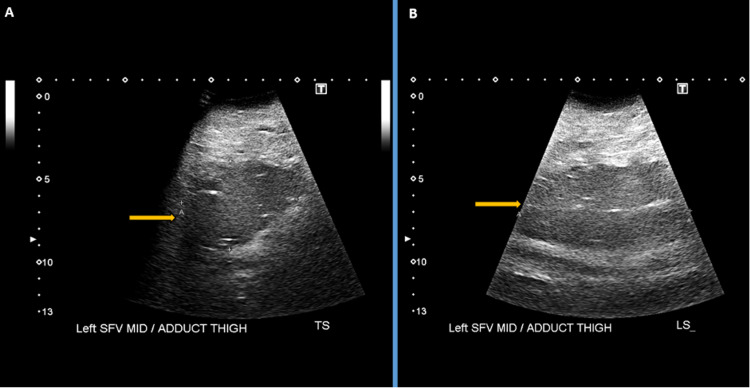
Pre-operative greyscale ultrasound transverse (A) and longitudinal (B) images showing a deep-seated intramuscular collection in the left mid-thigh represented by orange arrows
